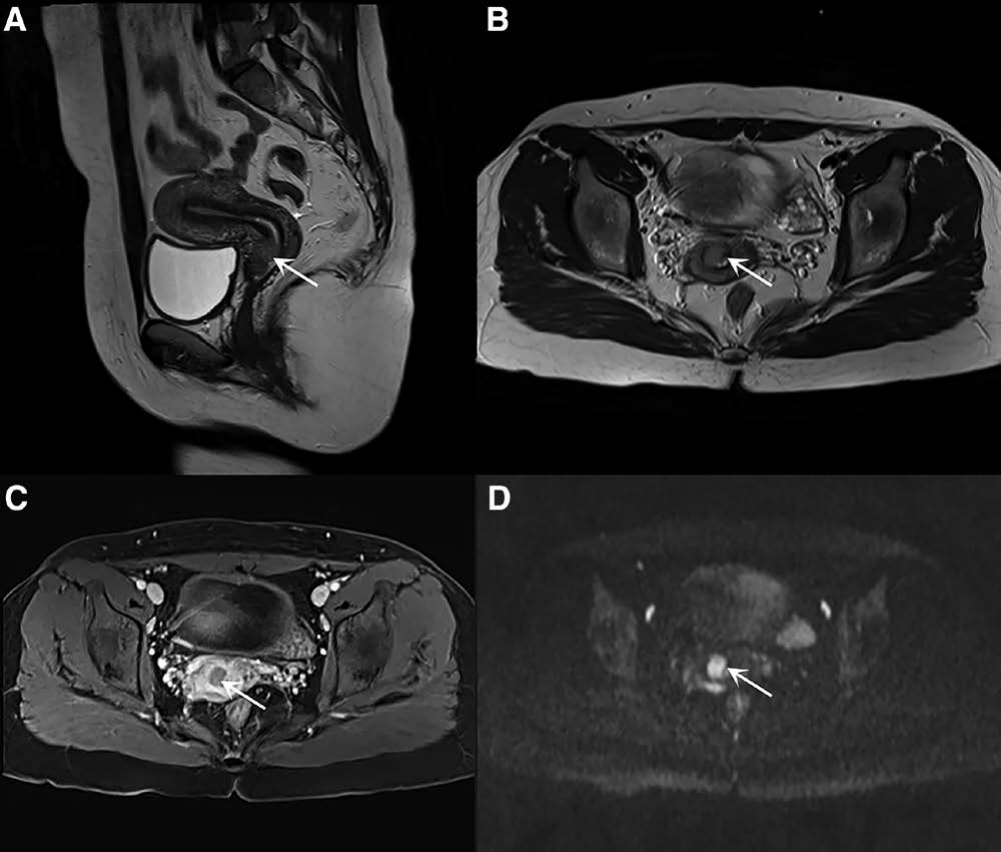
MRI of lymphoepithelioma-like carcinomas (LELCs) of the cervix (clock-wise A, B, C, D) (A, B) Sagittal (A) and axial (B) T2-weighted images show a focal mass (arrow) in the anterior lip of the exocervix. (C) Contrast-enhanced axial T1-weighted image shows a hypovascular cervical mass (arrow). (D) Diffusion-weighted image at b = 1000 s/mm^2^ shows hyperintensity (arrow) in the cervical mass. MRI = magnetic resonance imaging.
